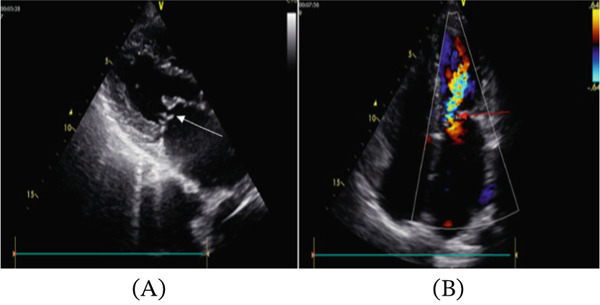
(A) Parasternal long‐axis view showing markedly thickened mitral valve leaflets with restricted diastolic opening (white arrow), consistent with severe rheumatic mitral stenosis. (B) Apical four‐chamber view with color Doppler demonstrating turbulent flow across the mitral valve (red arrow), indicating significant transmitral gradient.
